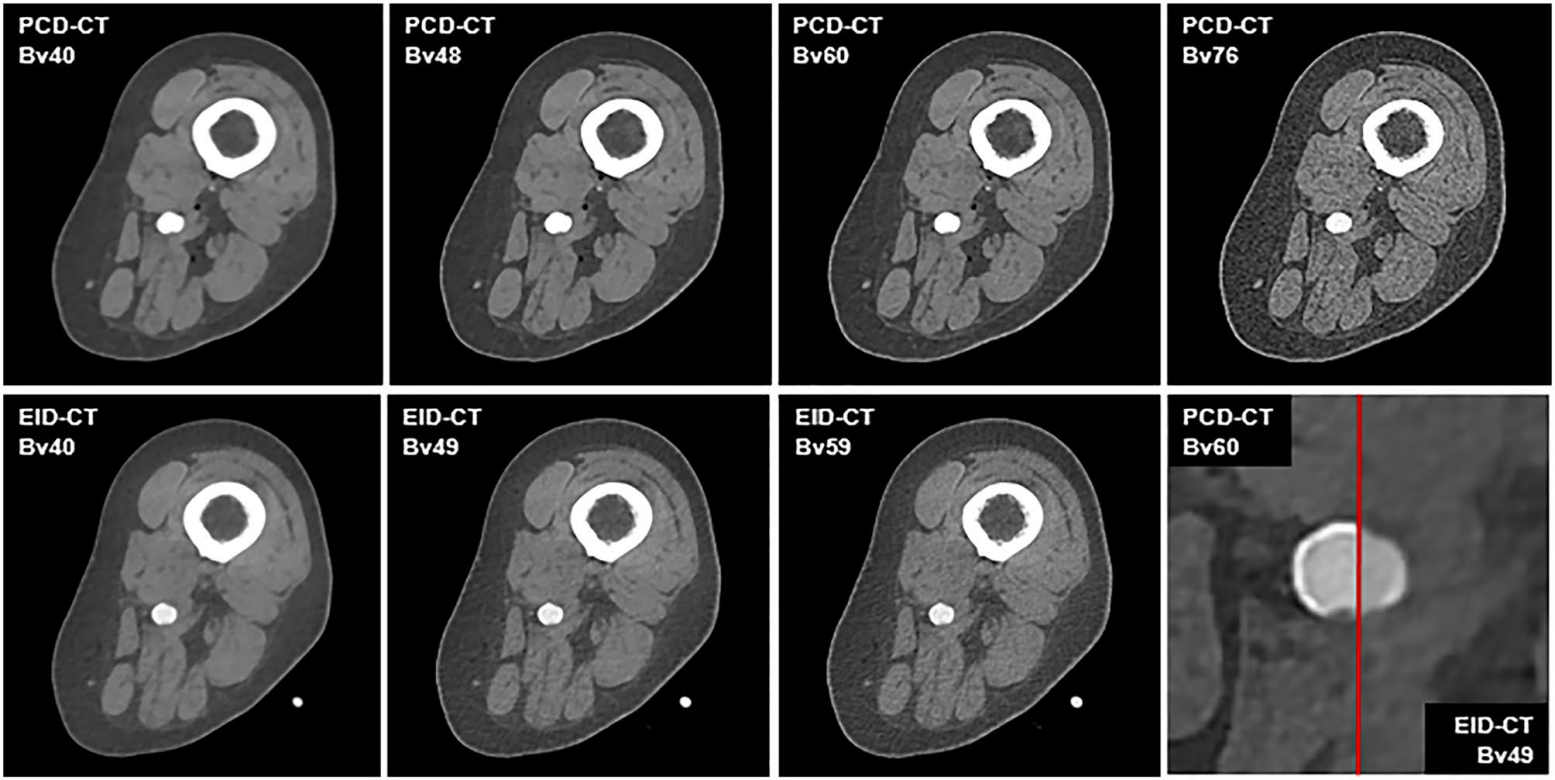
Image quality comparison between photon-counting and energy-integrating-detector CT. *Upper row* Photon-counting detector CT (PCD-CT). *Lower row* Energy-integrating detector CT (EID-CT). *Lower right corner* Direct comparison of a sharp kernel PCD-CT image (Bv60; left half) and a medium-sharp kernel EID-CT image (Bv49; right half) with equivalent SNR/CNR values in the same individual.
